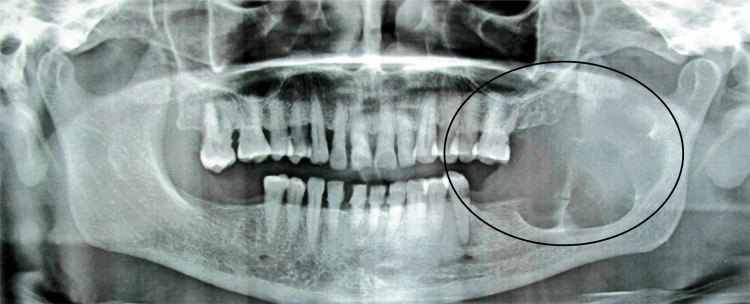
The orthopantomogram of the patient showing well-defined multilocular radiolucency on the left side of the mandible
